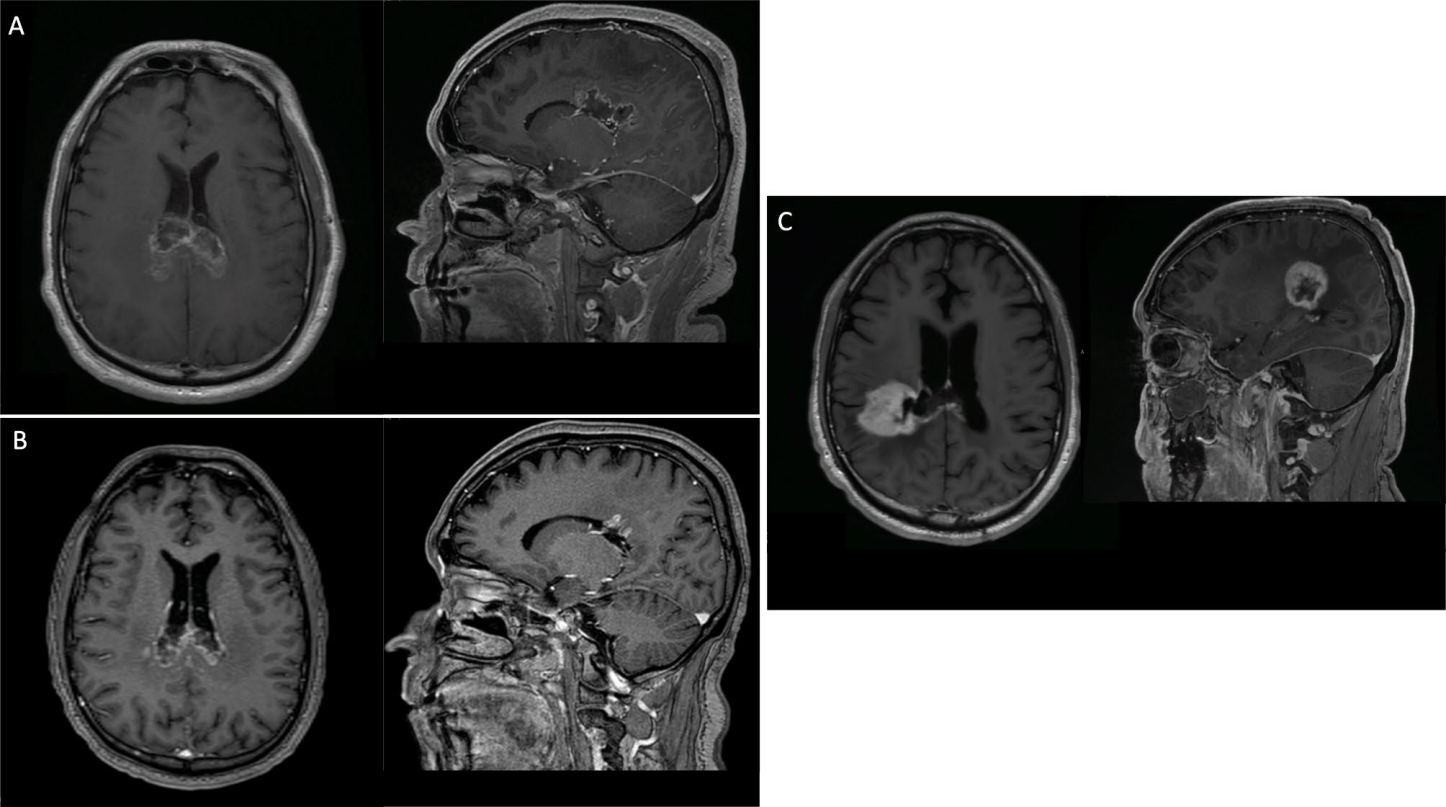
MRI brain T1-post contrast axial and sagittal views for a selected patient (A) 24-hours post-op prior to receiving radiosurgery with GammaKnife ICON, (B) one month after completion of radiation treatment showing a partial response per RECIST criteria, and (C) 3 years after completion of radiation treatment at time of radiographic evidence of disease progression
